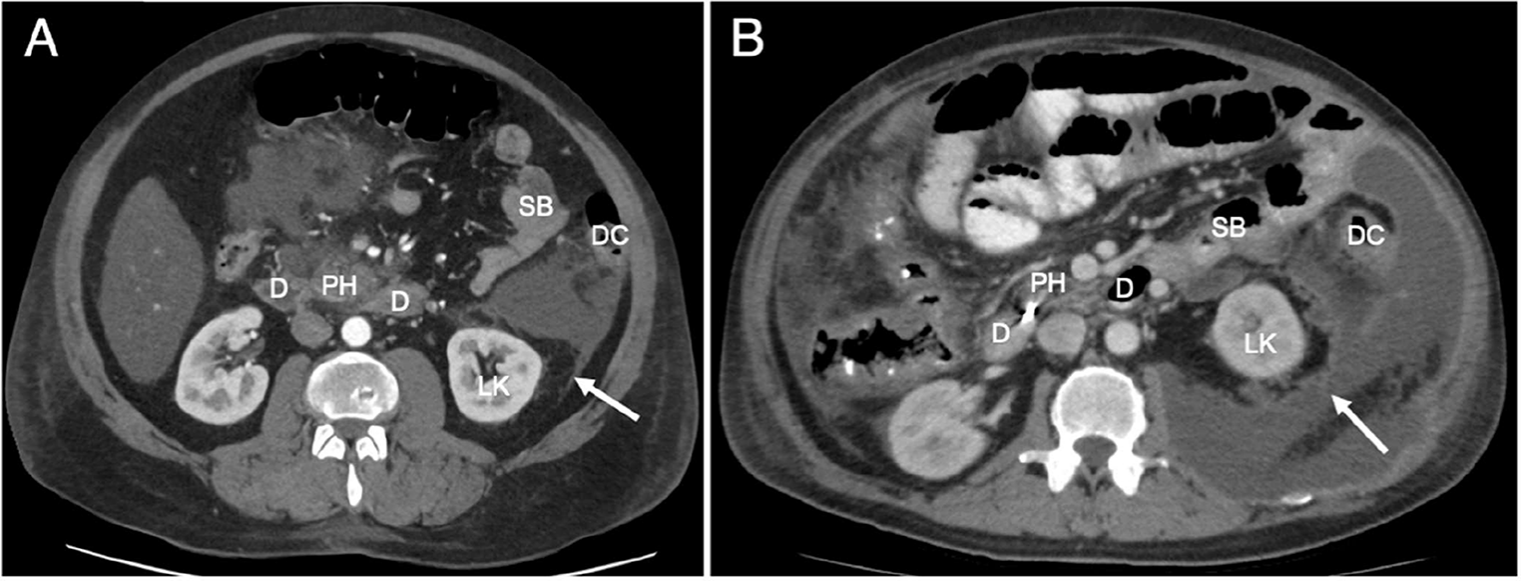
Imaging examples in CT of fluid tracking posteriorly along the renal fascia in two patients with pancreatitis. A) Fluid starting to spread posterior along the posterior lamina of the renal fascia (arrow). B) Massive amount of fluid spreading posteriorly around the renal fascia (arrow) extending medially all the way to the psoas major muscle D: duodenum, PH: pancreatic head, SB: small bowel, DC: descending colon, LK: left kidney
